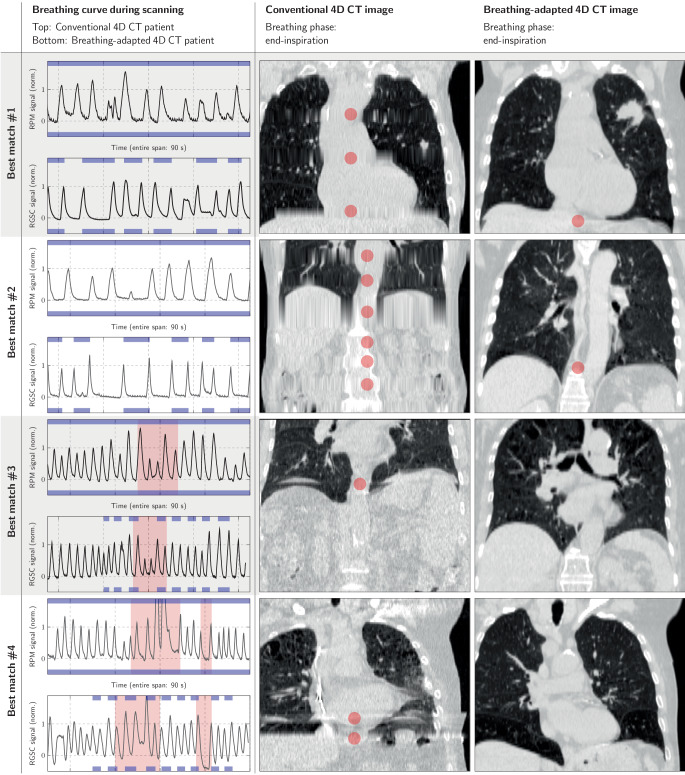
Comparison of the breathing curves and the corresponding end-inspiration images for the four ‘best matching’ i4DCT and conventional 4D CT patients with pronounced breathing irregularities but similar breathing patterns. For the breathing curves, the *top panel* shows the curve of the Hamburg case and the *bottom panel* the curve of the Erlangen cohort. The presented period is restricted to 90 s for all cases to ensure comparability. Note that the Erlangen curve was the reference curve for which we sought the best-fitting Hamburg curve (based on visual inspection). The intervals highlighted in *blue* indicate the beam-on periods during scan time (continuous for conventional spiral 4D CT; discontinuous intervals for breathing-adapted 4D CT (i4DCT). The *red blocks* for matches #3 and #4 highlight periods with pronounced breathing amplitude irregularity. The *red spots* in the image data indicate positions of pronounced image artifacts
